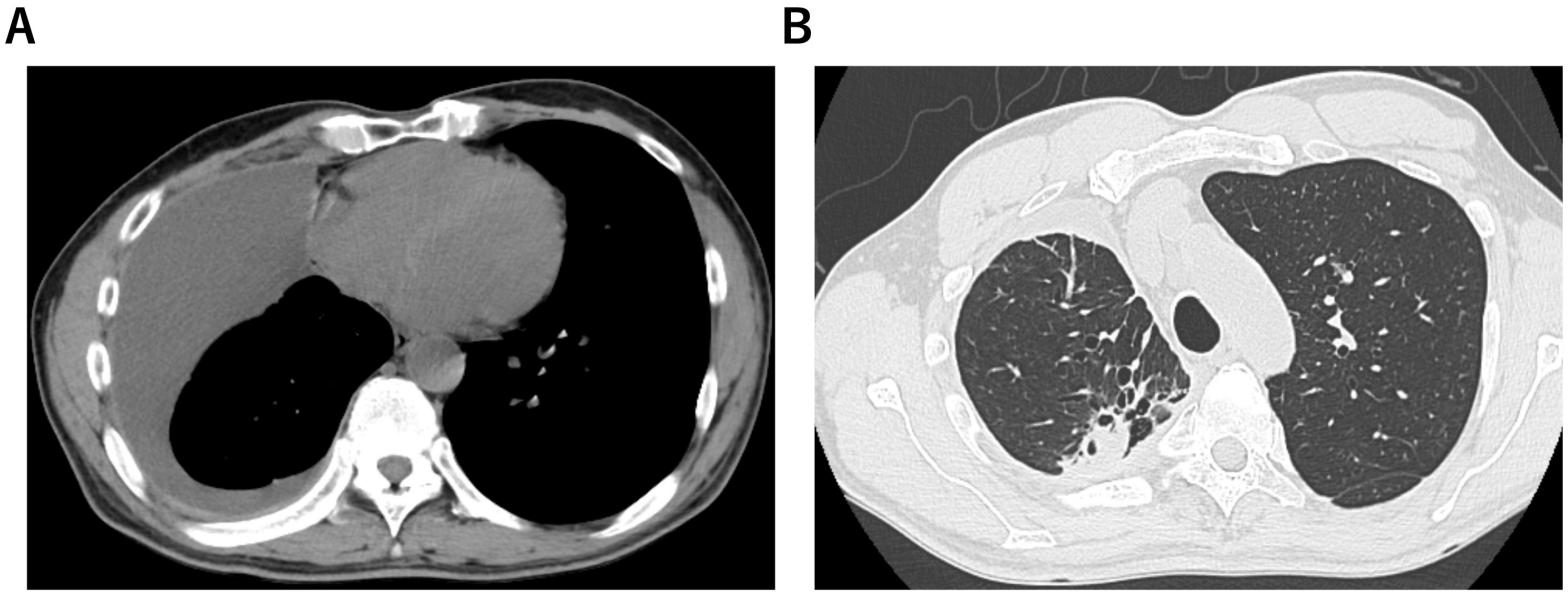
A patient with epidermal growth factor receptor-mutated non-small cell lung carcinoma. Computed tomography scans showed right pleural effusion (A) and nodules in the right upper lobe (B) before treatment with amivantamab.
